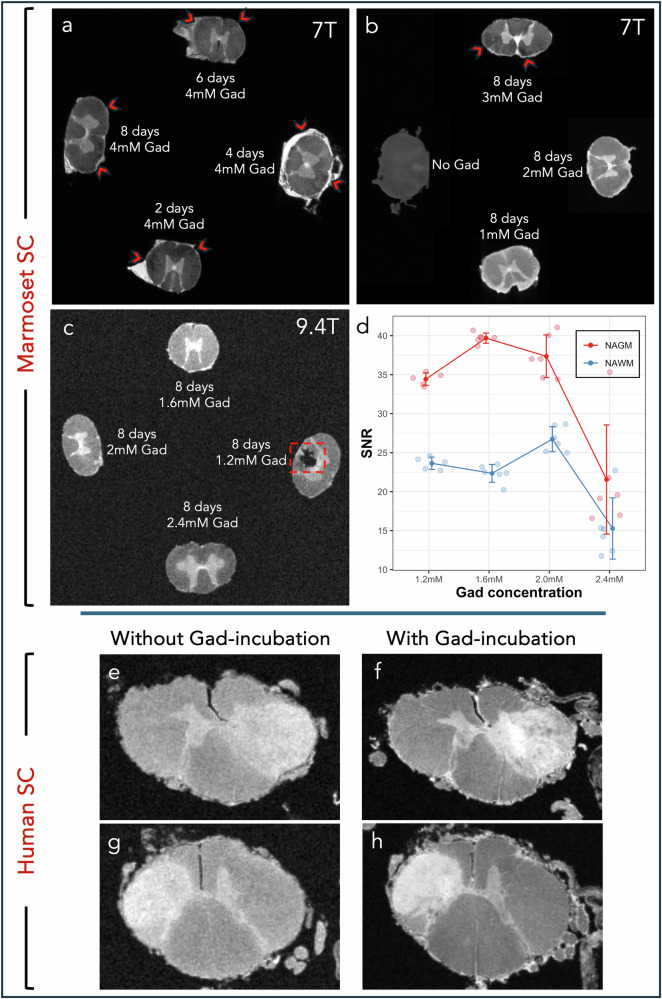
Optimization of gadolinium exposure for marmoset EAE SC tissue. a The four SC pieces of one EAE marmoset (EAE1) were immersed in a gadolinium solution (Gad) with a concentration of 4 mM for 2, 4, 6, and 8 days and then scanned at 7 T. b Next, the four SC pieces of a second EAE marmoset (EAE2) were immersed in a gadolinium solution with concentrations of 0, 1, 2, and 3 mM for 8 days and then scanned at 7 T. Gadolinium concentrations ≥3 mM led to signal inhomogeneities in the marmoset EAE SC (a, b; red arrowheads). c The four SC pieces of a third EAE marmoset (EAE3) were immersed in a gadolinium solution with concentrations of 1.2, 1.6, 2, and 2.4 mM for 8 days and then scanned at 9.4 T. The red dashed square shows a SC hemorrhagic lesion detected in EAE3. d Mean normal-appearing gray and white matter (NAGM; NAWM) signal-to-noise ratio (SNR) ± standard deviation of SC tissue scanned at 9.4 T and incubated at various Gad concentrations (number of samples for each tissue type and Gad concentration = 6). Comparison of postmortem T2*w MRI of human SC before (e–g) and after (f–h) incubation in 1.6 mM gadolinium in phosphate-buffered saline (Gad-incubation) for 8 days, showing improved visualization of structural and pathological features and enhanced contrast between SC compartments. e, g MRI without Gad-incubation was acquired using a 3D T_2_*-weighted multi-echo gradient echo sequence with an in-plane resolution of 75 × 75 μm^2^, a slice thickness of 100 μm, and 48 averages. f, h MRI after Gad-incubation was acquired using a 3D T_2_*-weighted gradient single-echo sequence with an isotropic resolution of 75 μm and 20 signal averages.
